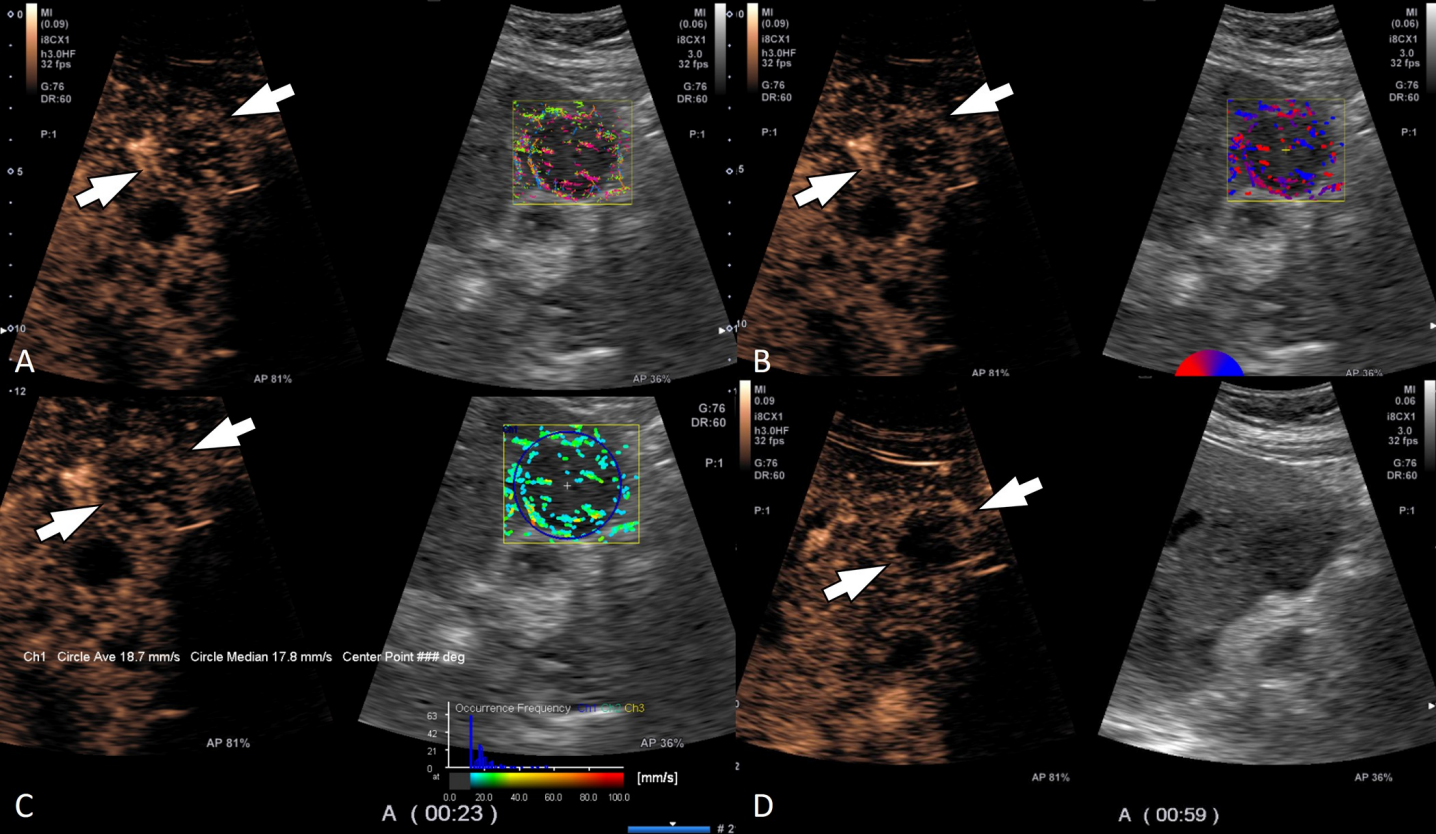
A 53-year-old woman with liver metastasis from GB cancer. (A) Contrast-enhanced ultrasound (left) and trace mode of contrast vector imaging (CVI) shows a peripheral rim pattern during the arterial phase. (B) Tumoral vascular structure was assessed as having both penetrating and detour patterns on velocity variance mode of CVI (right). (C) Histogram analysis showed low velocity less than 60 mm/s and the mean velocity was 18.7 mm/s. (D) The lesion showed early washout within 1 min.
